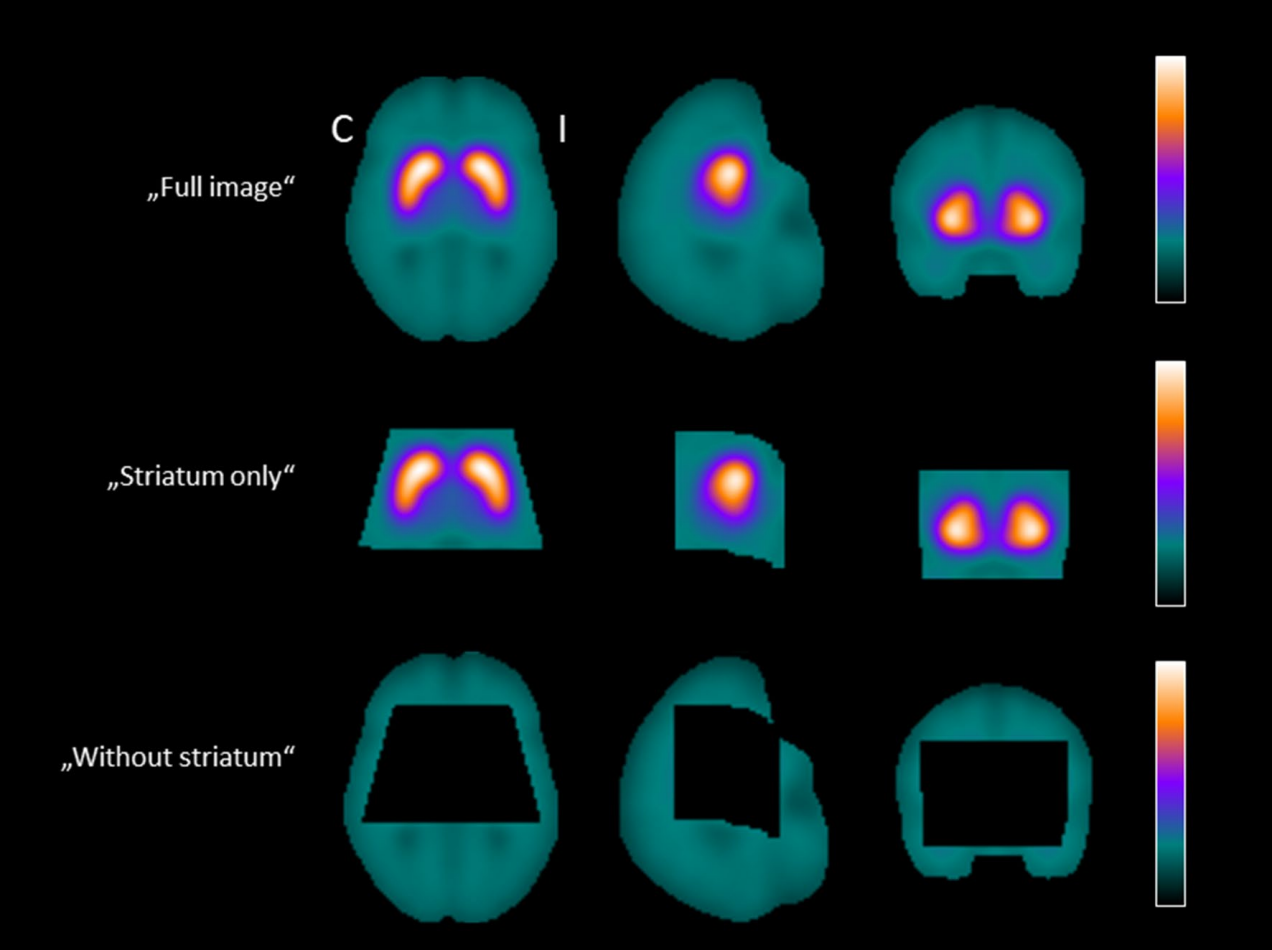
Settings for the CNN training: (i) full 3-dimensional ^123^I-FP-CIT SPECT images (“full image”), (ii) the 3-dimensional region of the striata cropped from the full image (“striatum only”), and (iii) full 3-dimensional ^123^I-FP-CIT SPECT with the region of the striata removed (“without striatum”). The mask of the striatal region used to generate the images for the “striatum only” and the “without striatum” settings was chosen with large safety margin around the striata in order to largely eliminate spill-out of striatal signal into the rest of the brain (*C* contralateral, *I* ipsilateral).
